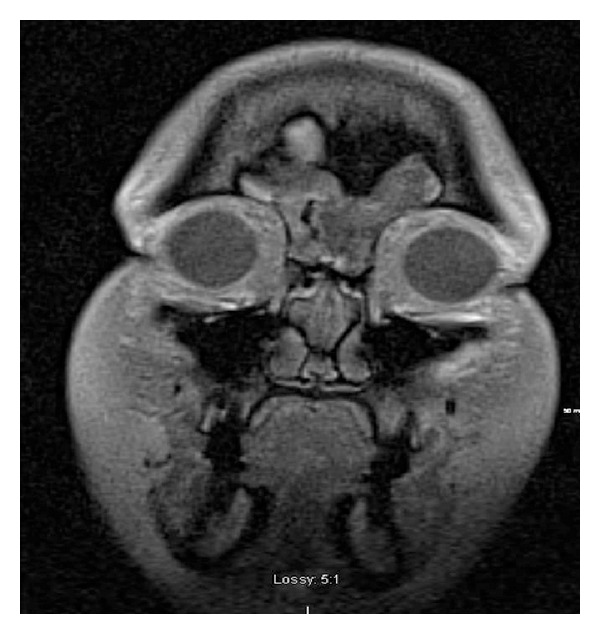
Coronal T1 weighted MRI showing the osseous mass filling the left frontal sinus and extending into the right.
